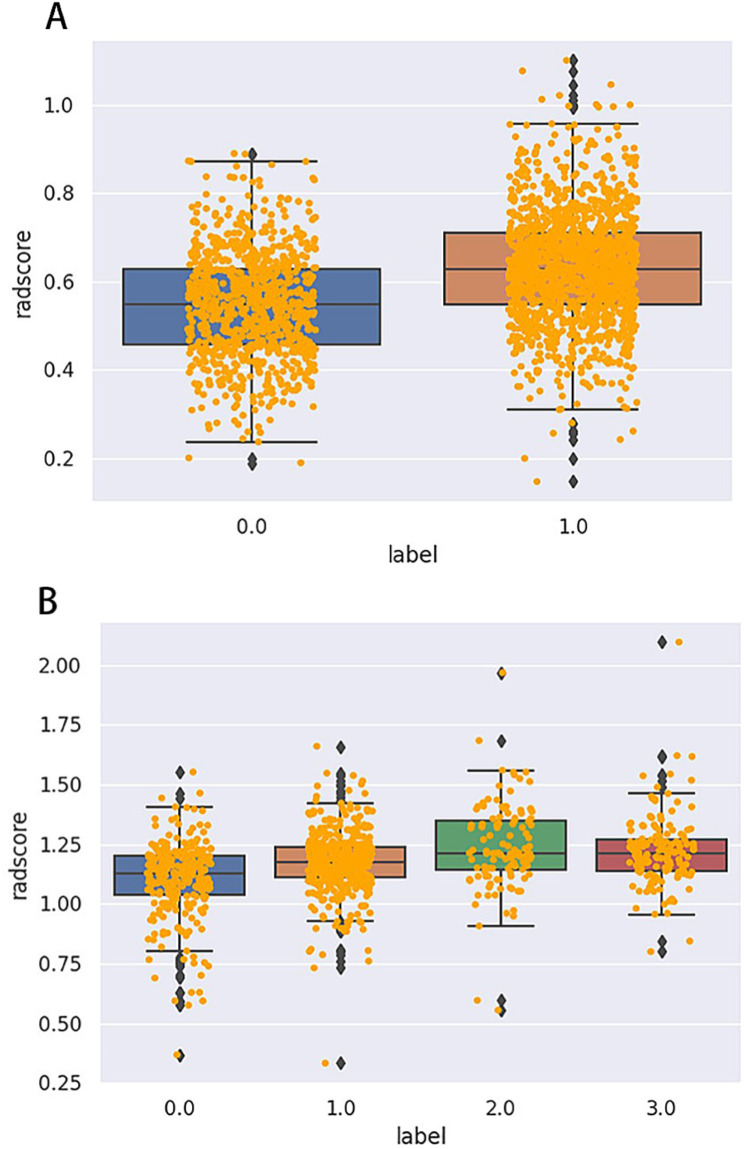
Radscores box plot of ultrasound image features for breast cancer postoperative recurrence risk (A) and molecular typing (B). The postoperative intermediate risk of recurrence was coded as 0, and the high risk of recurrence was coded as 1 (A). The HER-2 overexpression type was coded as 0, TNBC as 1, luminal A as 2, and luminal B as 3 (B). A total of 733 features were extracted from the ultrasound images of the patients. After normalizing the extracted features, we get Radscores. First, we find the upper edge, lower edge, median, and two quartiles of Radscores. Then, we connect the two quartiles to draw a box. Then, we connect the upper and lower edges to the box, and the median is in the middle of the box. The yellow dots represent the extracted features, and the blue diamonds represent outliers. In the figure, the median is in the middle of the box, and the data is normally distributed
